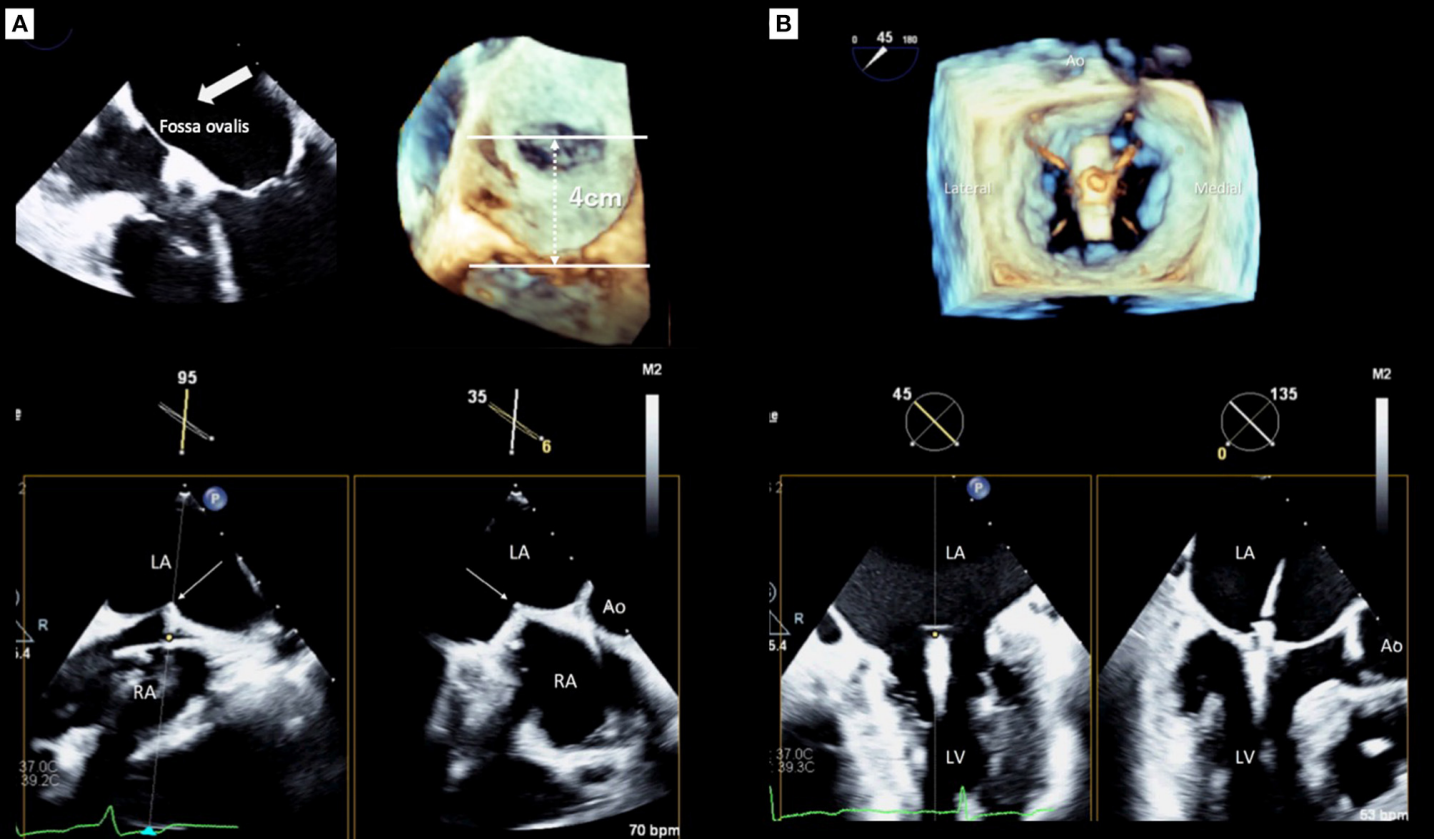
Real-time guidance for MitraClip implantation using three-dimensional transesophageal echocardiography. (A) Transseptal puncture guidance, (B) Clip deployment. LA, left atrium; LV, left ventricle; Ao, aorta.
